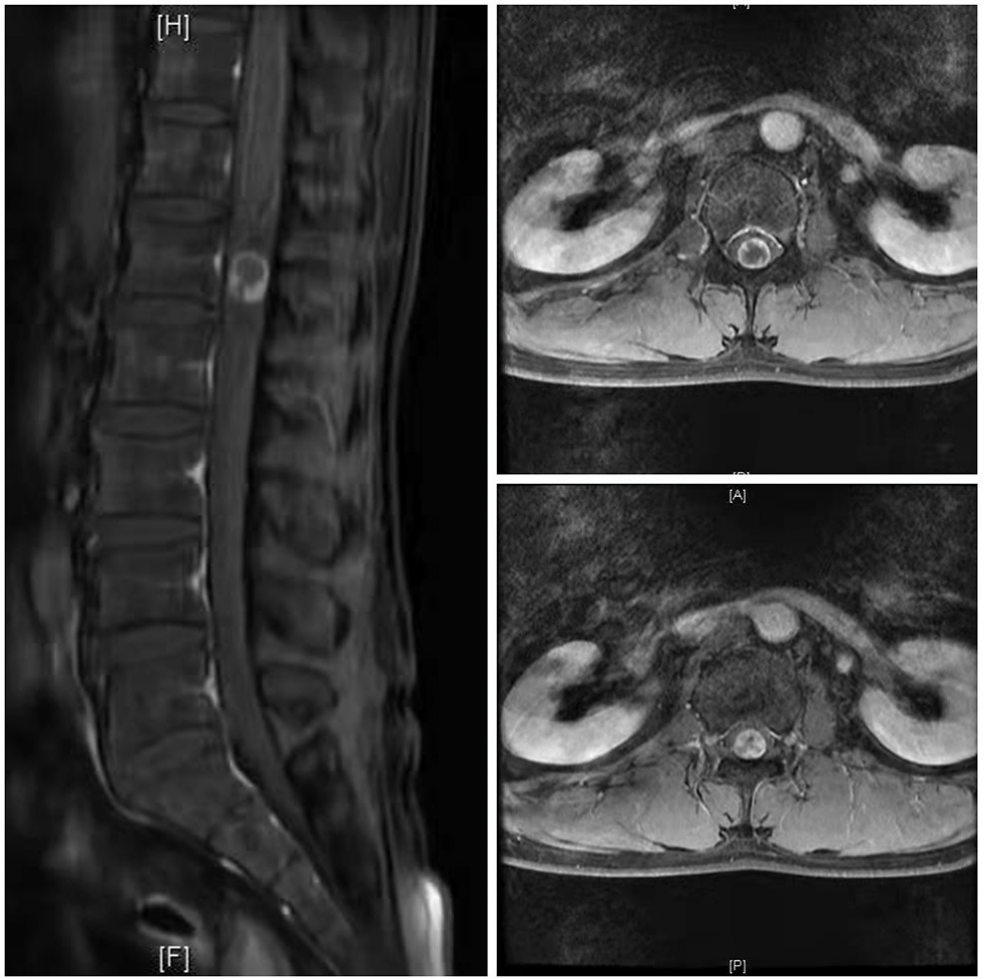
Preoperative MRI of the case 1.
